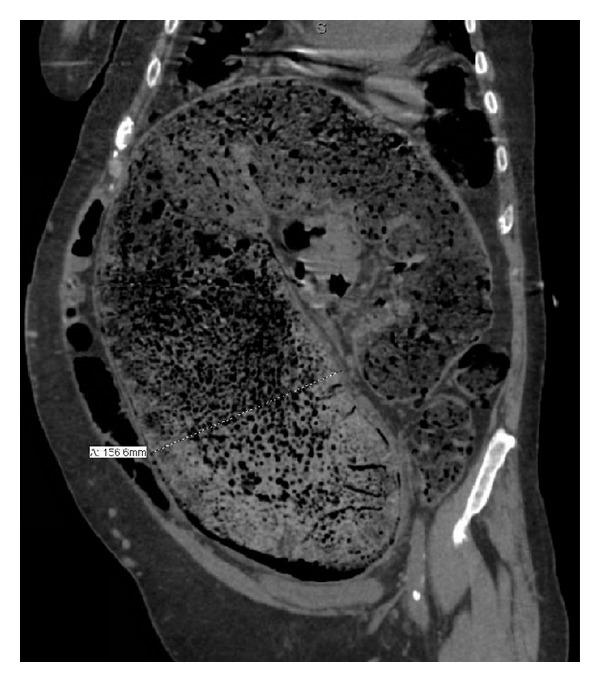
Coronal section on a computerized tomography (CT) scan showing severe fecal impaction. In this particular section, the rectum is noted to be distended up to approximately 16 centimeters.
